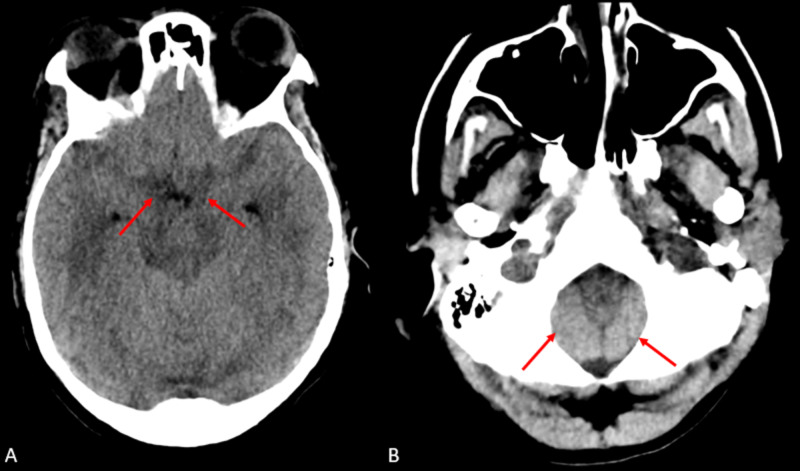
Axial CT head without contrast on presentation (A) There is intermediate density within the suprasellar cistern which could be secondary to exudates from meningitis (red arrows). (B) There is crowding of the foramen magnum with low-lying cerebellar tonsils (red arrow) CT: computed tomography
